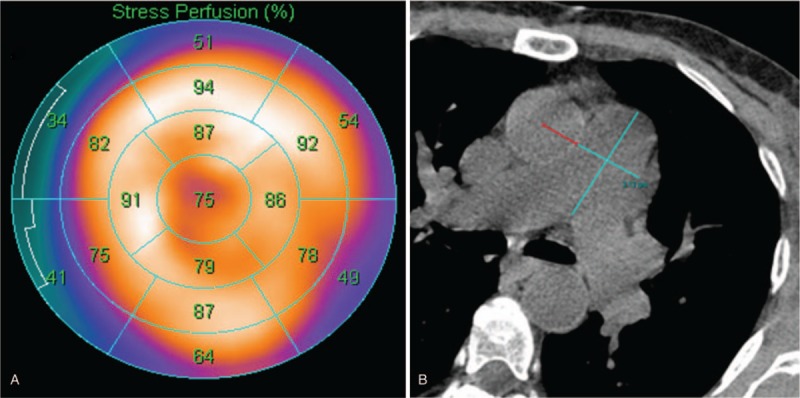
^99m^Tc sestamibi stress myocardial perfusion map (A) and axial gated-computed tomography (CT) image (B) of the main pulmonary artery of a 60-year-old hypertensive woman who underwent a ^99m^Tc sestamibi myocardial perfusion scintigraphy as part of a preoperative cardiac evaluation for a liver transplantation. The myocardial perfusion map during stress demonstrates normal myocardial perfusion with no regions of decreased radiotracer distribution. The axial gated-CT image demonstrates a dilated main pulmonary artery, measuring 31.3 mm.
